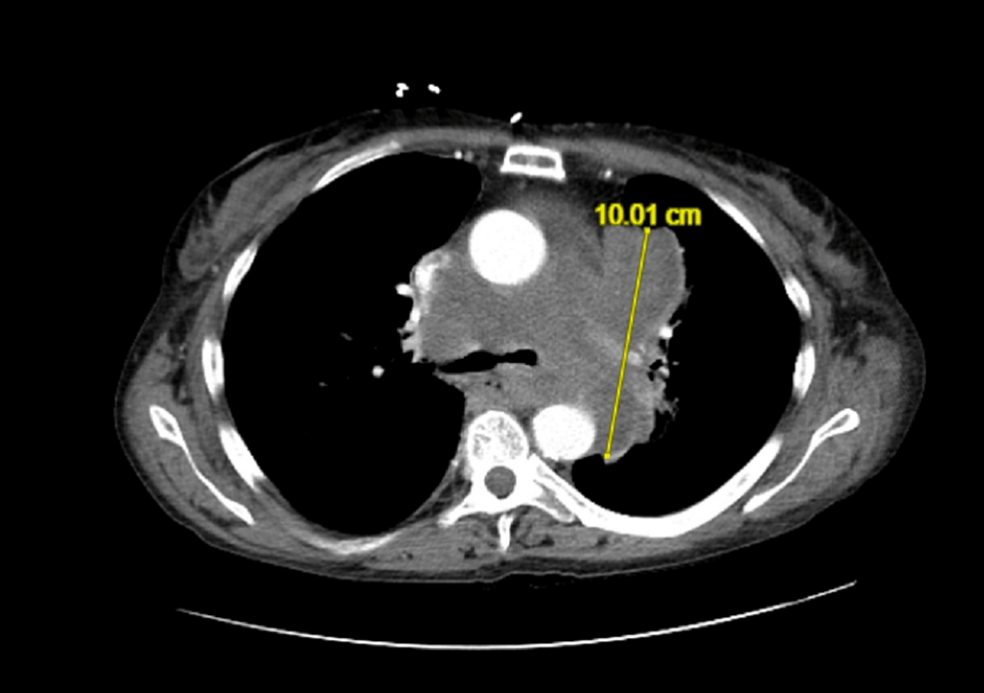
CT chest with IV contrast
